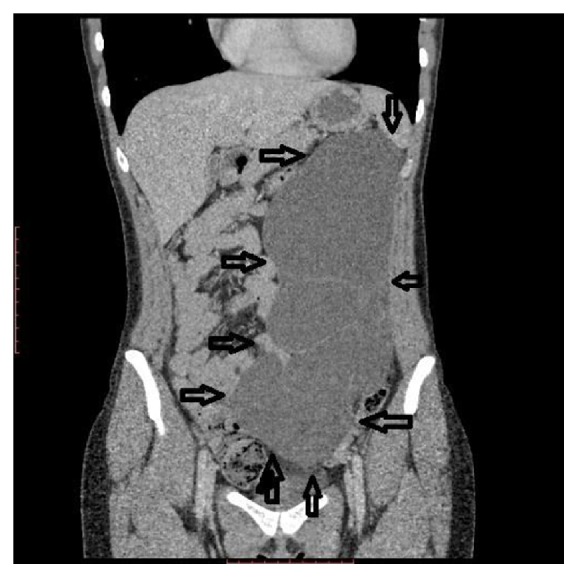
CT abdomen, coronal plane showing huge cystic retroperitoneal lymphangioma.
